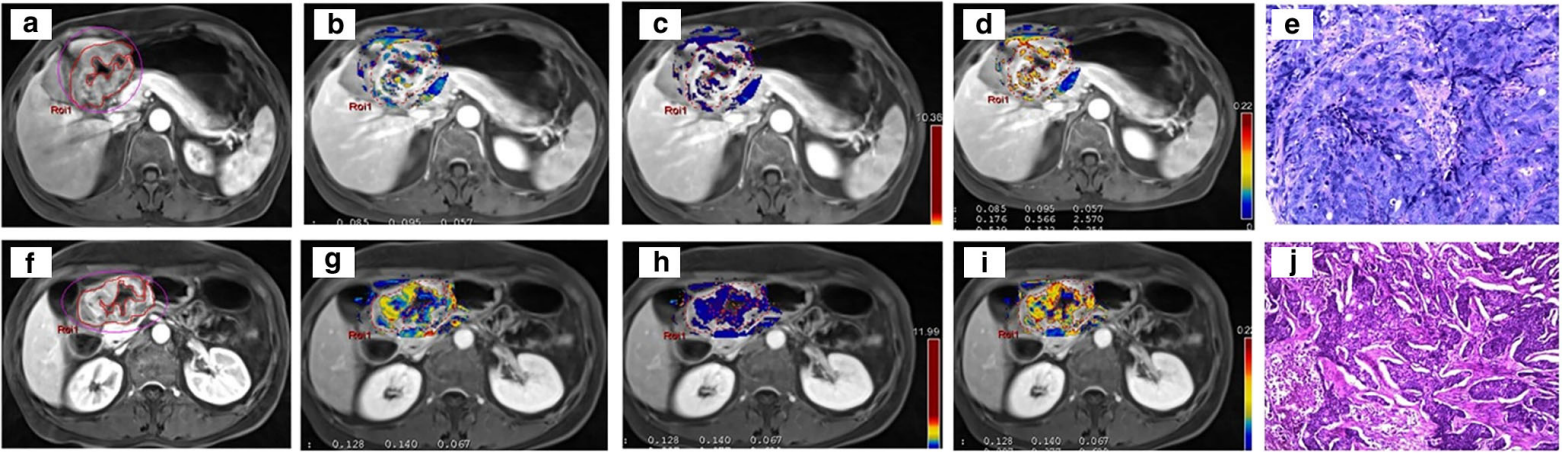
DCE-MRI of a tumour of the gastric antrum (a) in a 61-year-old female. In the pretreatment scan, the K^trans^ (b) was 0.085 min^−1^, the K_ep_ (c) was 0.176 min^−1^ and the V_e_ (d) was 0.539. The tumour was confirmed at biopsy (e). In the posttreatment scan, the tumour is still visible (f), and the K^trans^ (g) was 0.128 min^−1^, the K_ep_ (h) was 0.297 min^−1^ and the V_e_ (i) was 0.455. Final pathology (j): diffuse type (Lauren classification), staged as ypT3N0 (tumour regression grade 3)
